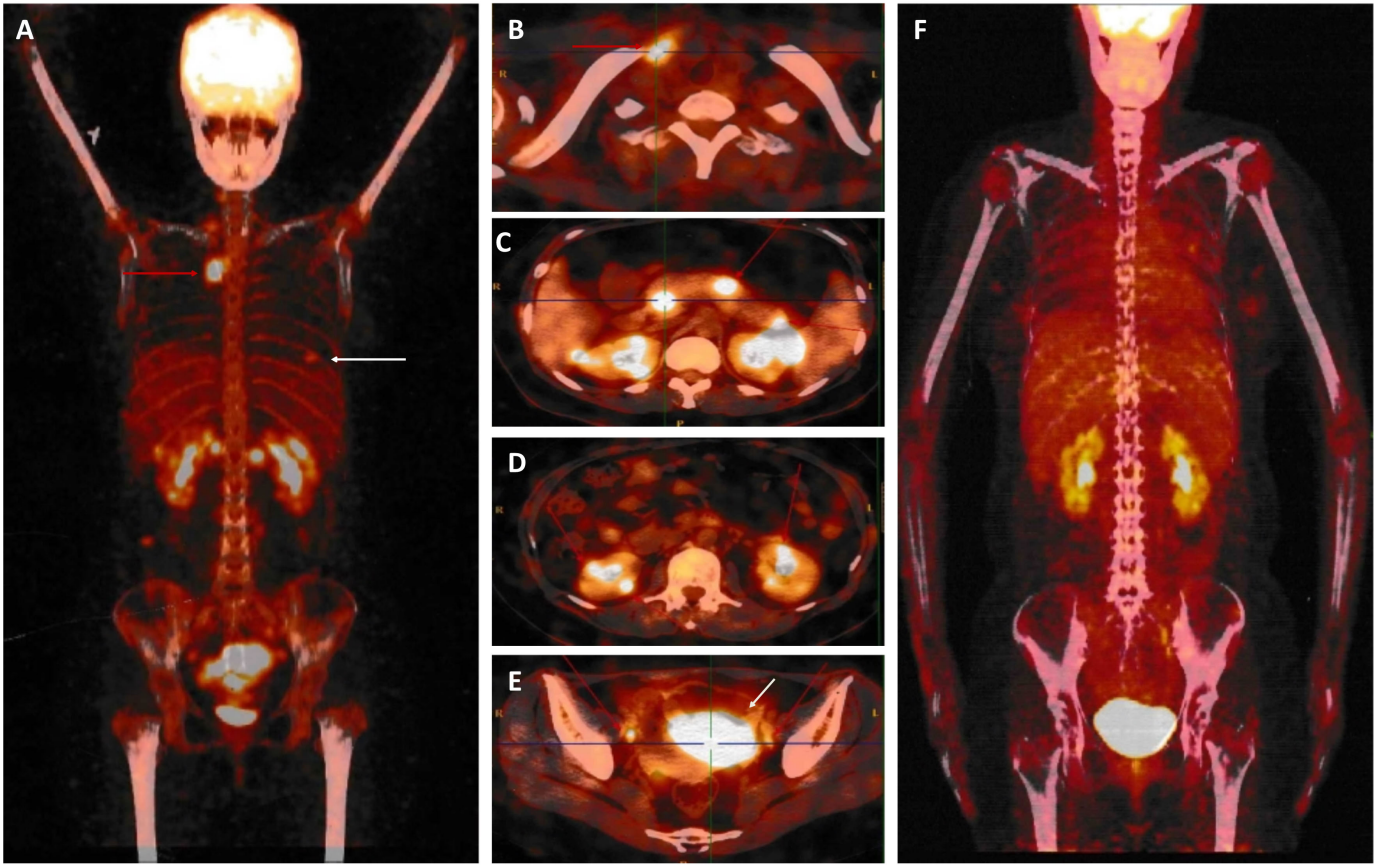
Whole body 18-fluorodeoxyglucose positron emission tomography-computed tomography. (A-E). Before starting treatment with CAR-T cell therapy, PET/CT data revealed abnormally intense high-metabolic masses in the breast [white arrow in (A)], lymph nodes [red arrow in (A, B, E)], pancreas (C), kidney (D), and uterus [white arrow in (E)]. (F). After CAR-T cell therapy, complete remission occurred.
